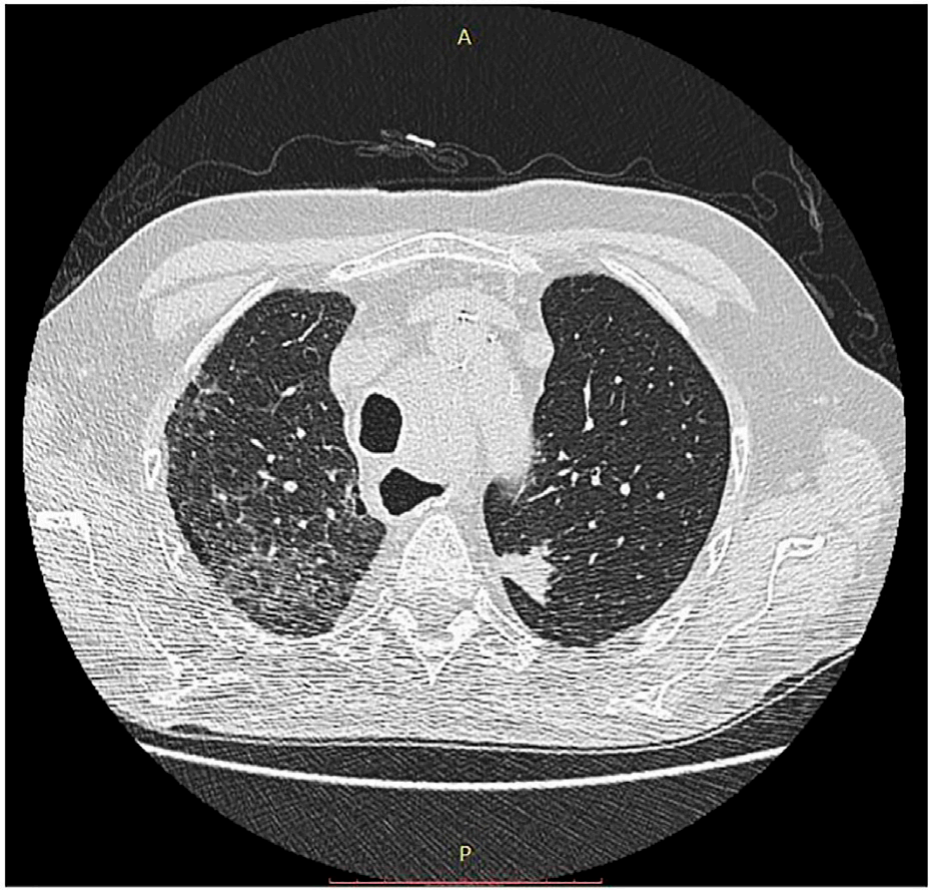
CT image of pneumonitis of pembrolizumab-treated cancer patient. With permission of Hungarian Society of Clinical Oncology (5).
